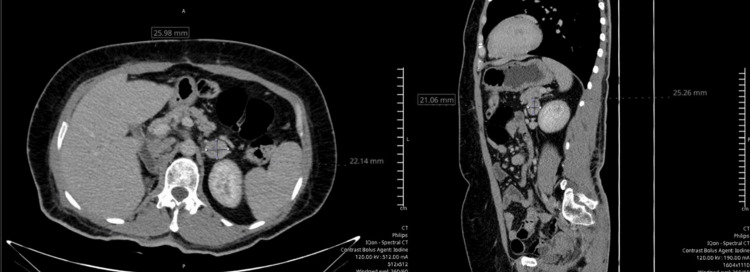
Contrast-enhanced CT demonstrating progressive left adrenal lesion Contrast-enhanced CT scan of the left adrenal gland (November 2023) demonstrating a lesion measuring 25 × 21 mm with central necrosis and 28% contrast washout. Compared with prior imaging in June 2023 (18 × 10 mm), the lesion shows progressive growth despite systemic therapy. Left panel: axial view. Right panel: sagittal view.
